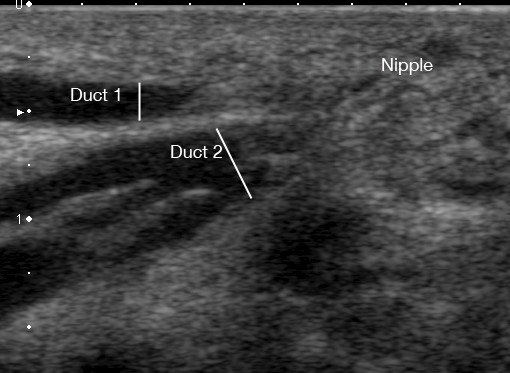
Ultrasound image of a milk ducts in the human lactating breast prior to milk ejection. Two main milk ducts are displayed on ultrasound as a hypoechoic (black) structure with echogenic walls (white). Duct 1 is more superficial (1.95 mm) than Duct 2 (3.72 mm). Duct 2 has three merging branches.
